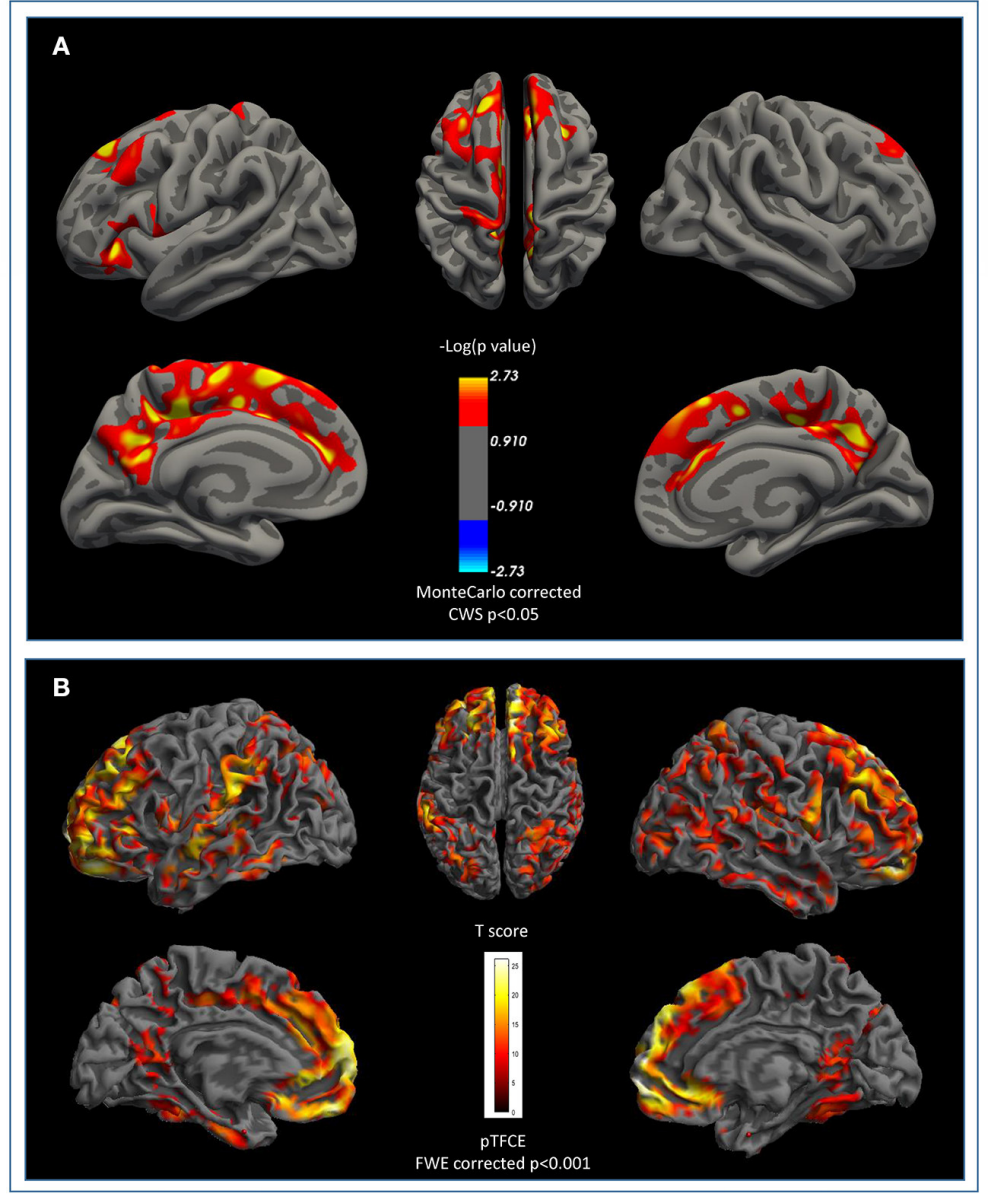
β-Amyloid cortical deposition and brain atrophy in PD-MCI. (A) Surface based comparison between standardized uptake value ratios (SUVR) in PD-MCI-Aβ+ vs. Aβ-. Areas that survived a cluster-wise Monte-Carlo correction (*p* < 0.05) adjusted for two-hemispheres are displayed. Cerebellum cortex was used as reference region for partial volume correction. (B) Shared pattern of atrophy between PD-MCI-Aβ+ vs. Aβ- compared to healthy matched population at FWE pTFCE *p* < 0.001. No areas survived after β-amyloid +/– at TFCE uncorrected *p* < 0.001 threshold. FWE, family-wise error; TFCE, Threshold-Free Cluster Enhancement.
